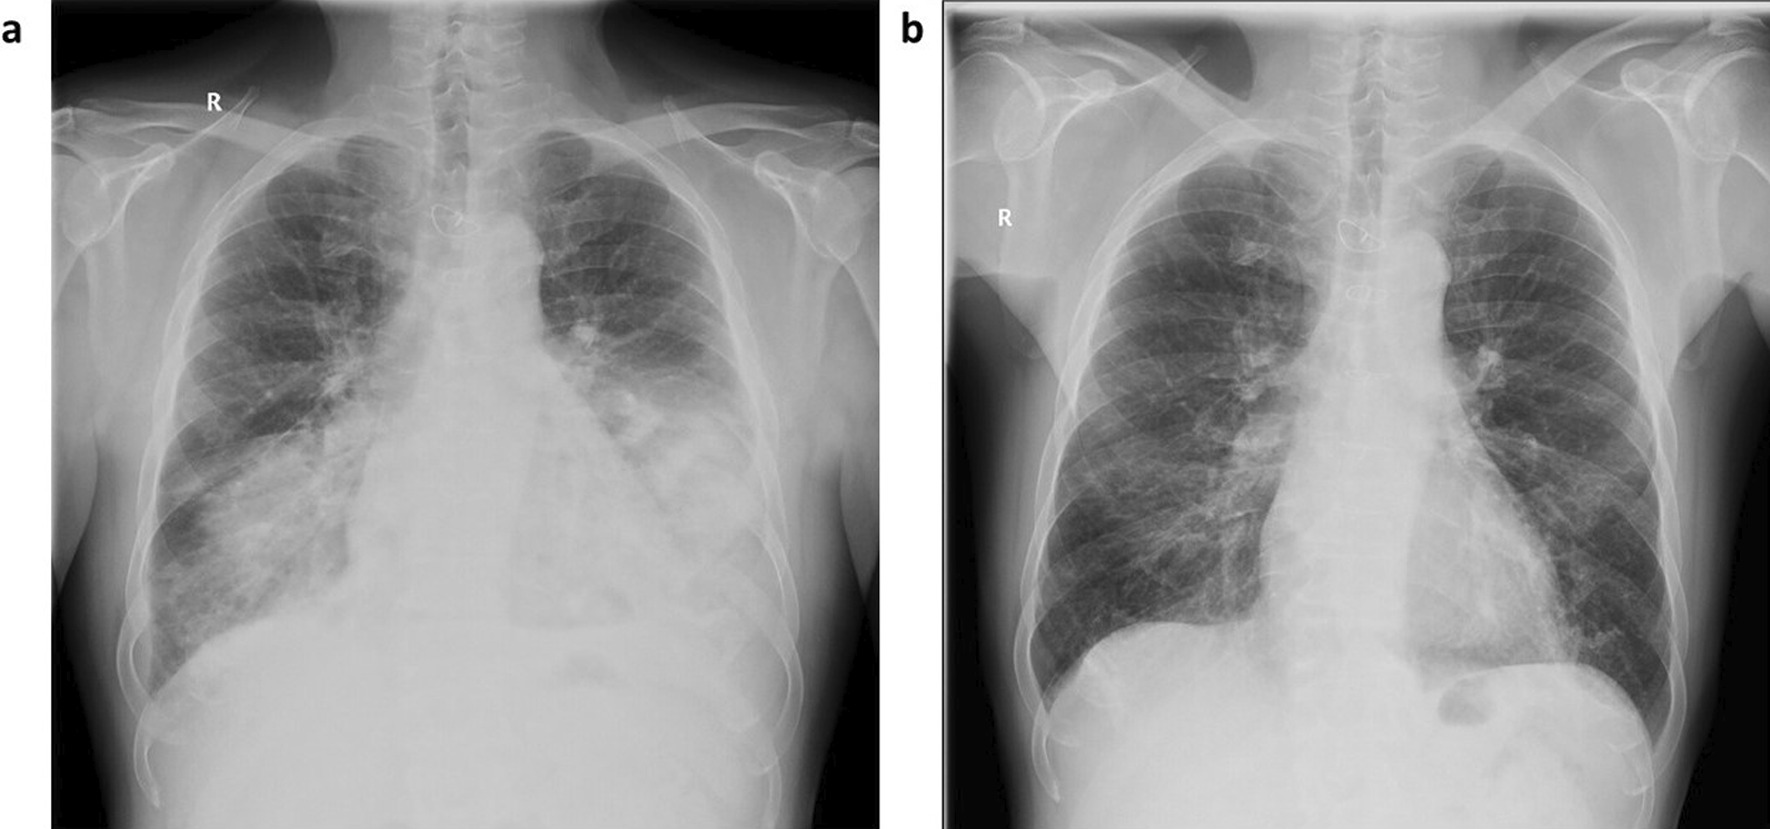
Chest X-ray. a at hospital admission showing extensive bilateral infiltrates predominantly in basal segments of the lungs; b two weeks after corticosteroid administration with almost complete resolution of bilateral lung infiltrates
